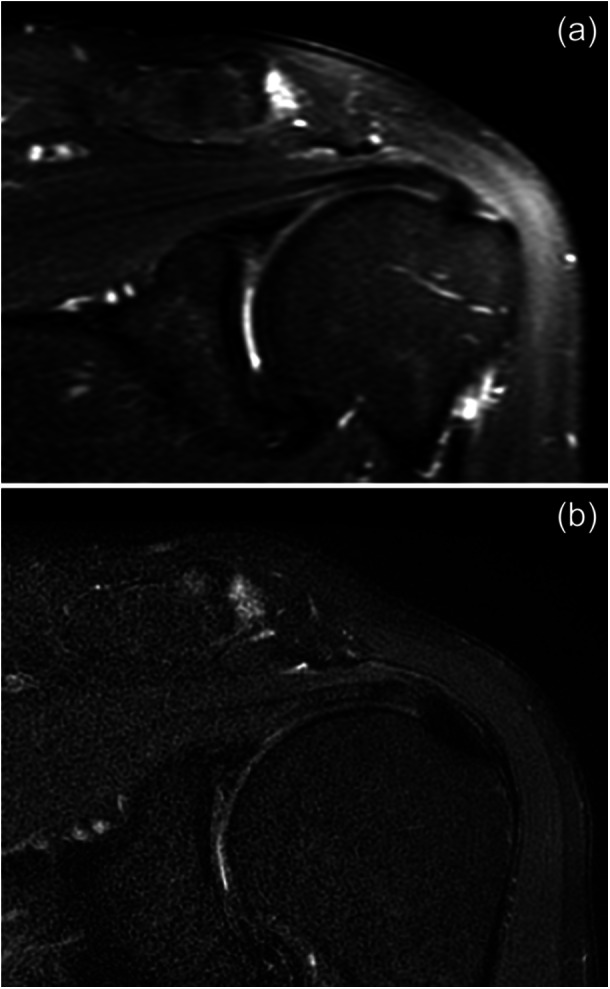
(a) Magnetic resonance image (MRI; coronal view T2) that shows a high‐grade partial bursal supraspinatus tear. (b) MRI image of the same patient 1 year after isolated Regeneten® implantation over the tear, showing complete healing of the tear and additional tendinous tissue healing up to the lateral greater tuberosity.
